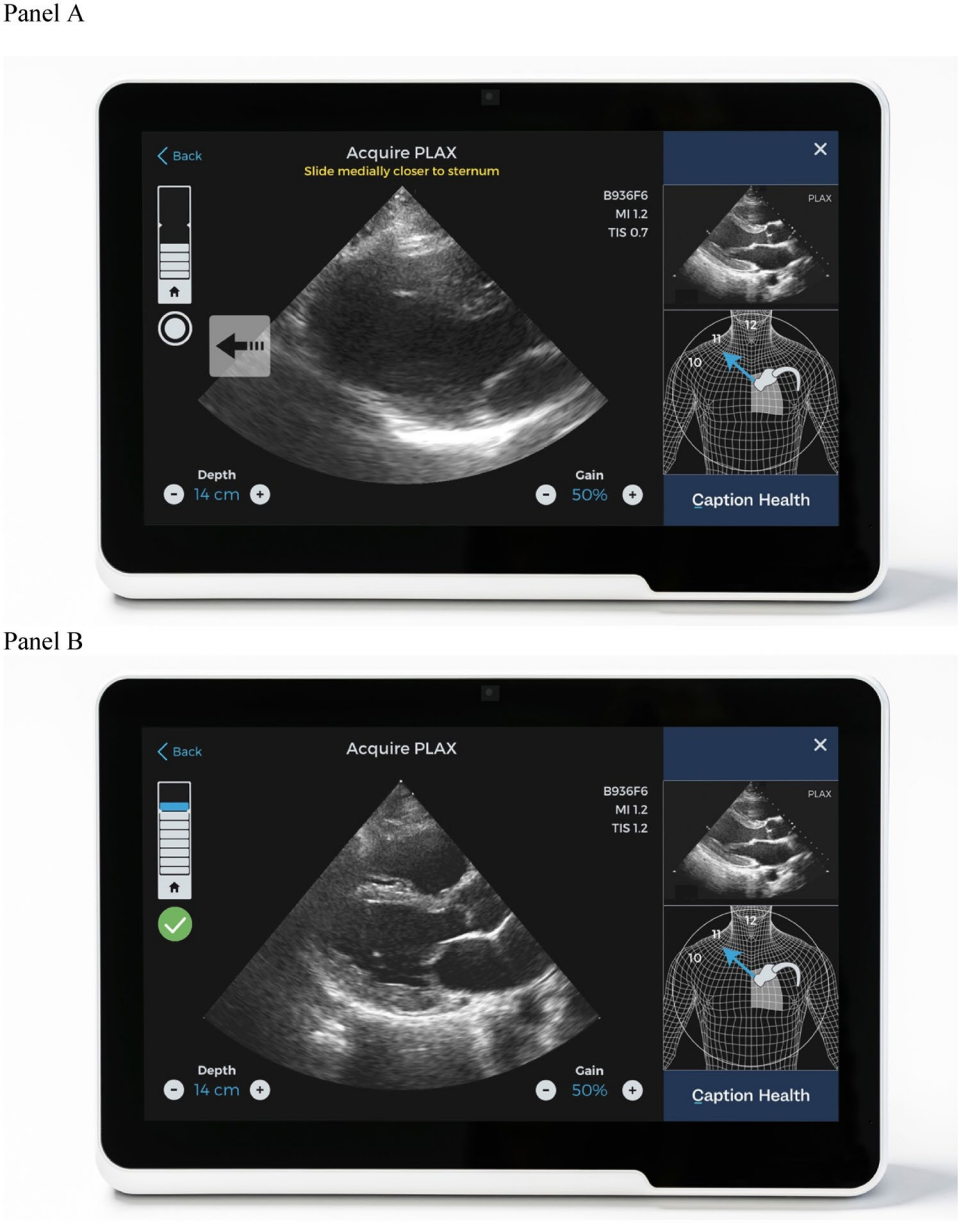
Panel a shows the guidance phase: The image is not optimal and the guidance message is shown (“slide medially closer to the sternum”). The quality meter shows that it has not reached the automated capture level. Panel b shows the screen when a good view has been obtained and automated capture has occurred. *PLAX* parasternal long axis view
